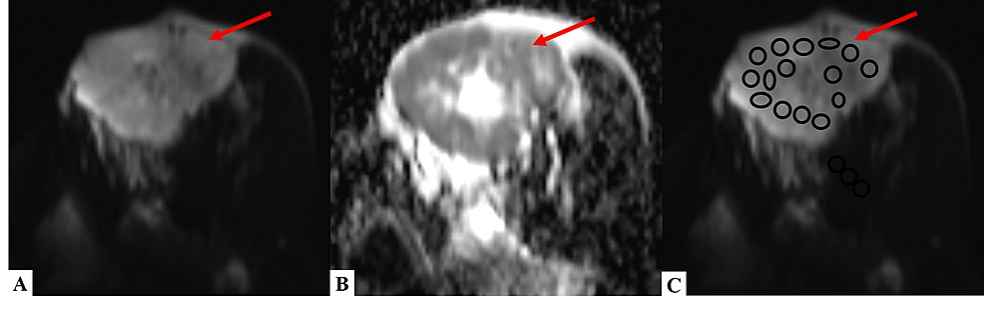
DWI and ADC sequences of a patient with infiltrative ductal carcinoma (A) DWI and (B) corresponding ADC images showing the peripheral area of restriction of diffusion within the lesion present in the left breast- suggestive of malignant etiology. (C) DWI image demonstrating the method of placing multiple ovoid ROIs (each measuring ~25 mm^2^) in the areas of restricted diffusion for calculation of mean ADC value. DWI - diffusion-weighted imaging; ADC - apparent diffusion coefficient; ROI - region of interest
